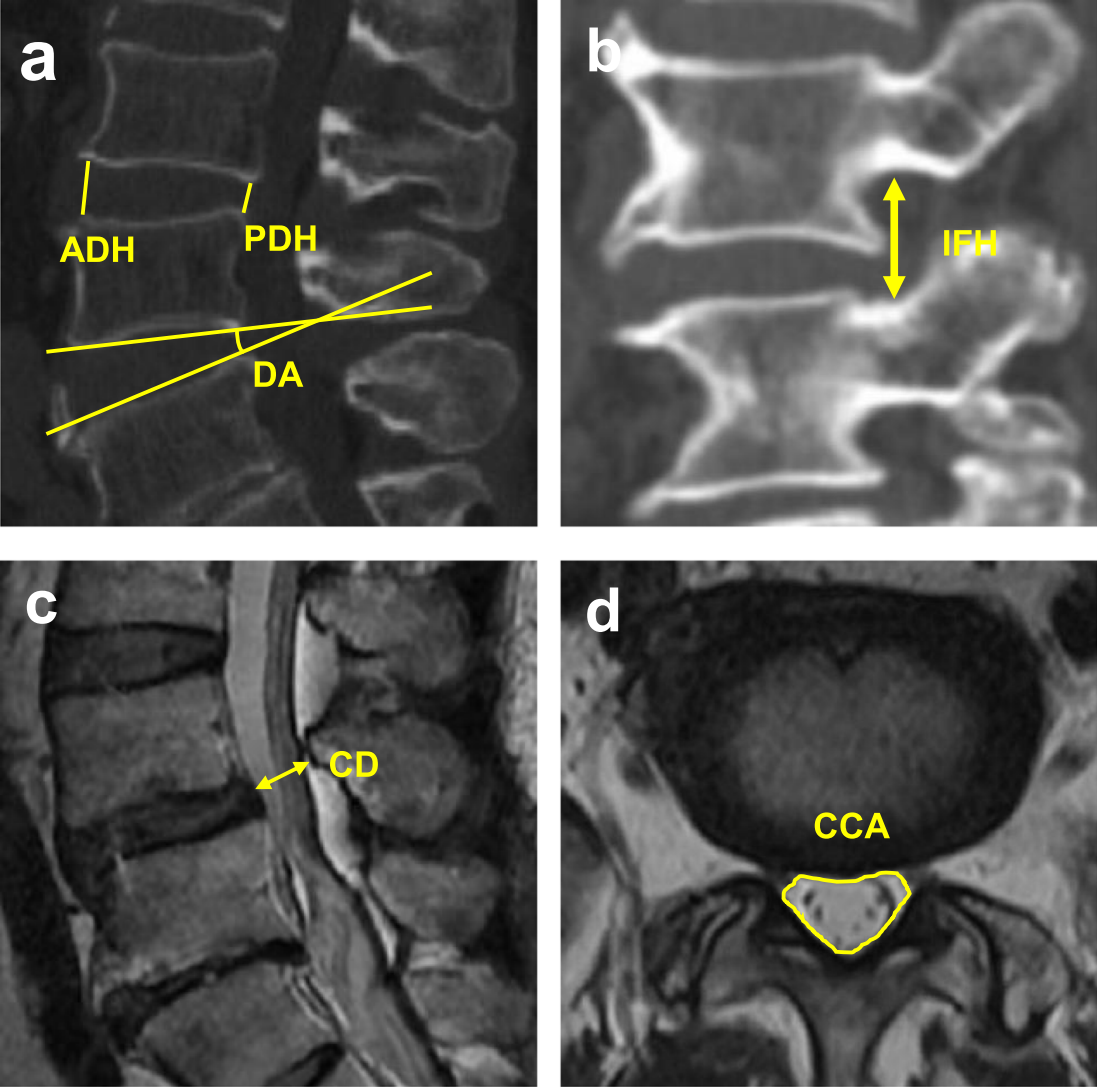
Measurement of radiographic parameters. ADH: anterior disc height. PDH: posterior disc height. DA: disc angle. IFH: intervertebral foramen height. CD: midsagittal canal diameter. CCA: axial central canal area
